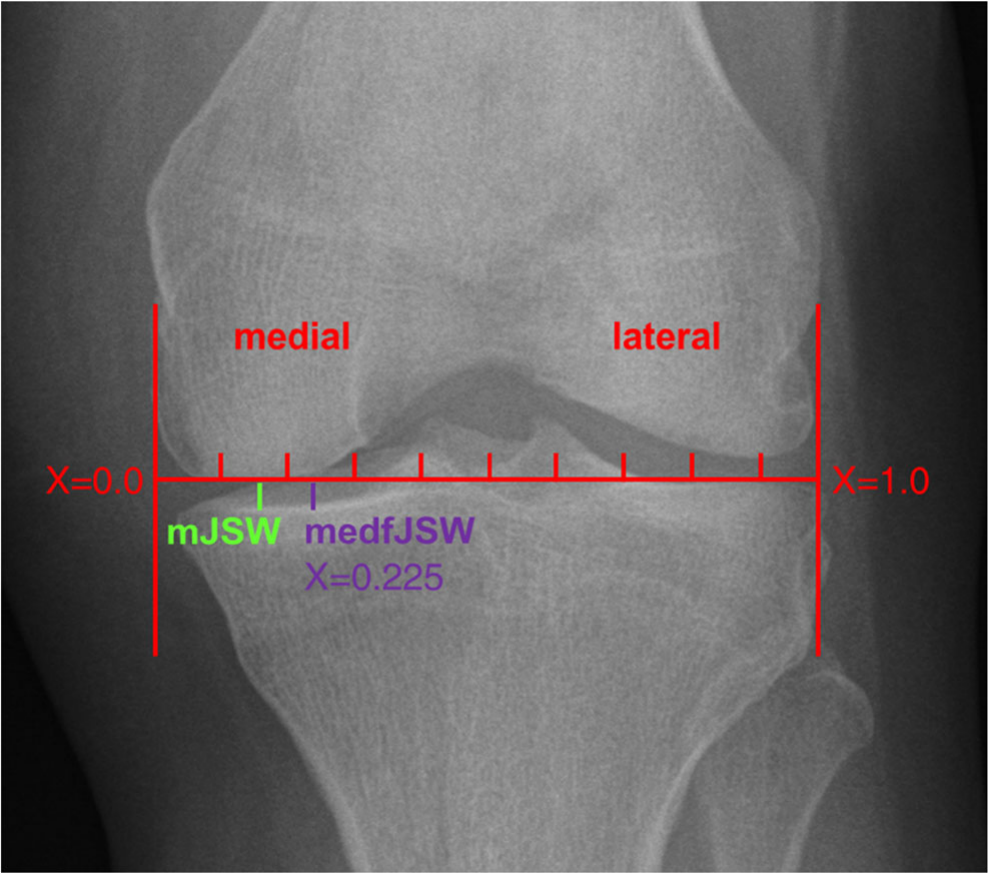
radiographic joint space width. Fixed-flexion radiograph depicting the medial to lateral coordinate system used for fixed-location measurement (medfJSW: medial fixed joint space width at 22.5%), as well as the minimum medial joint space width (mJSW)
